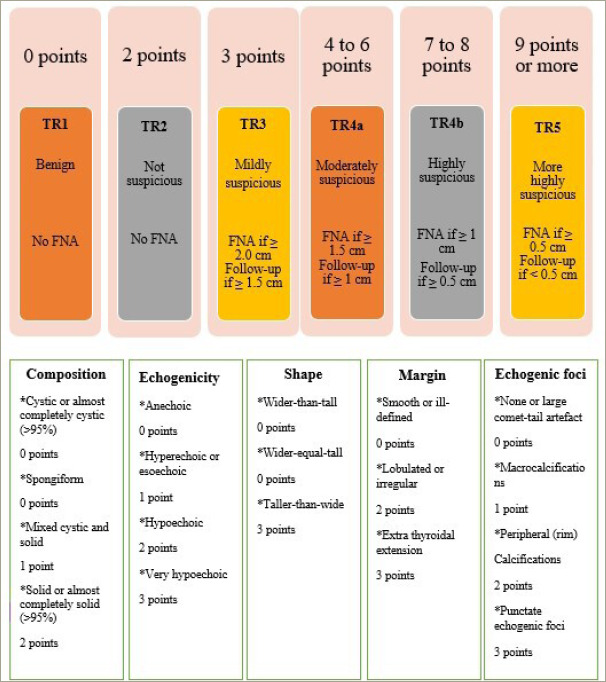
Six categories of the revised thyroid imaging reporting and data system, thyroid imaging reporting and data system levels and criteria for fine-needle aspiration or follow-up ultrasound^24^
